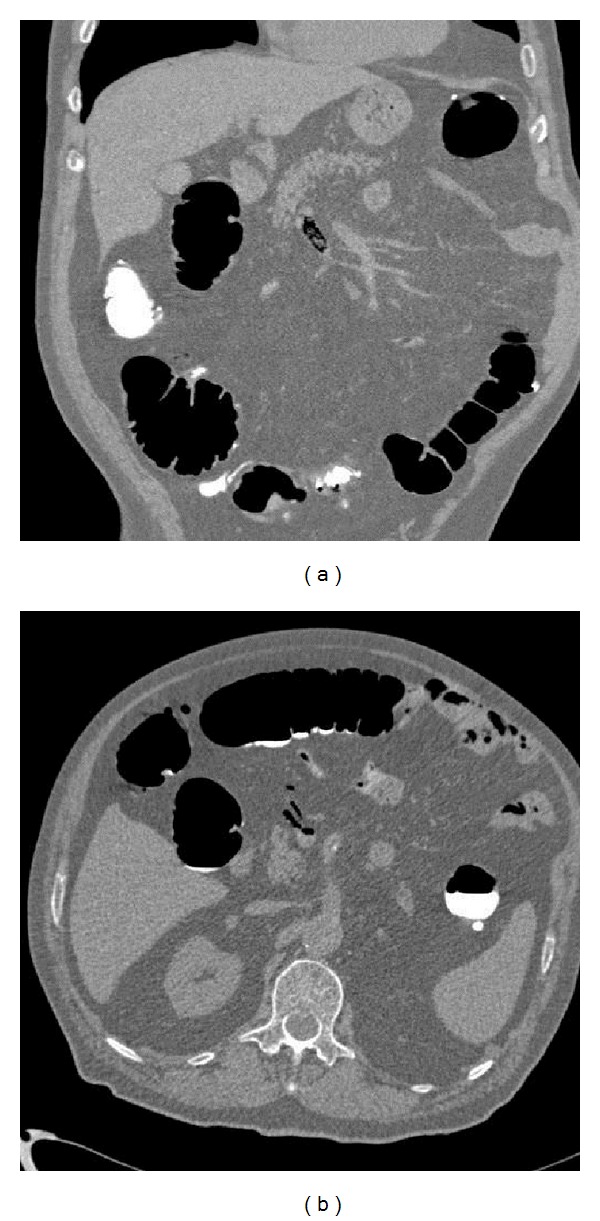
(a) Coronal contrast enhanced CT of the abdomen demonstrated the presence of gas in mesenteric vein and in the portal venous system. (b) Axial contrast enhanced CT of the abdomen demonstrated the presence of gas in mesenteric vein and in the portal venous system.
